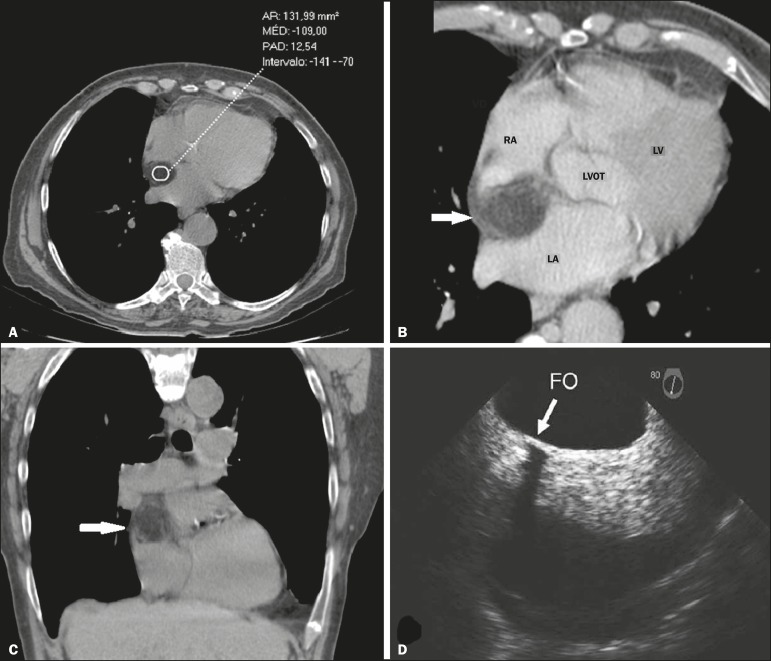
A: Non-contrast-enhanced CT scans showing IAS thickening of 2.4
